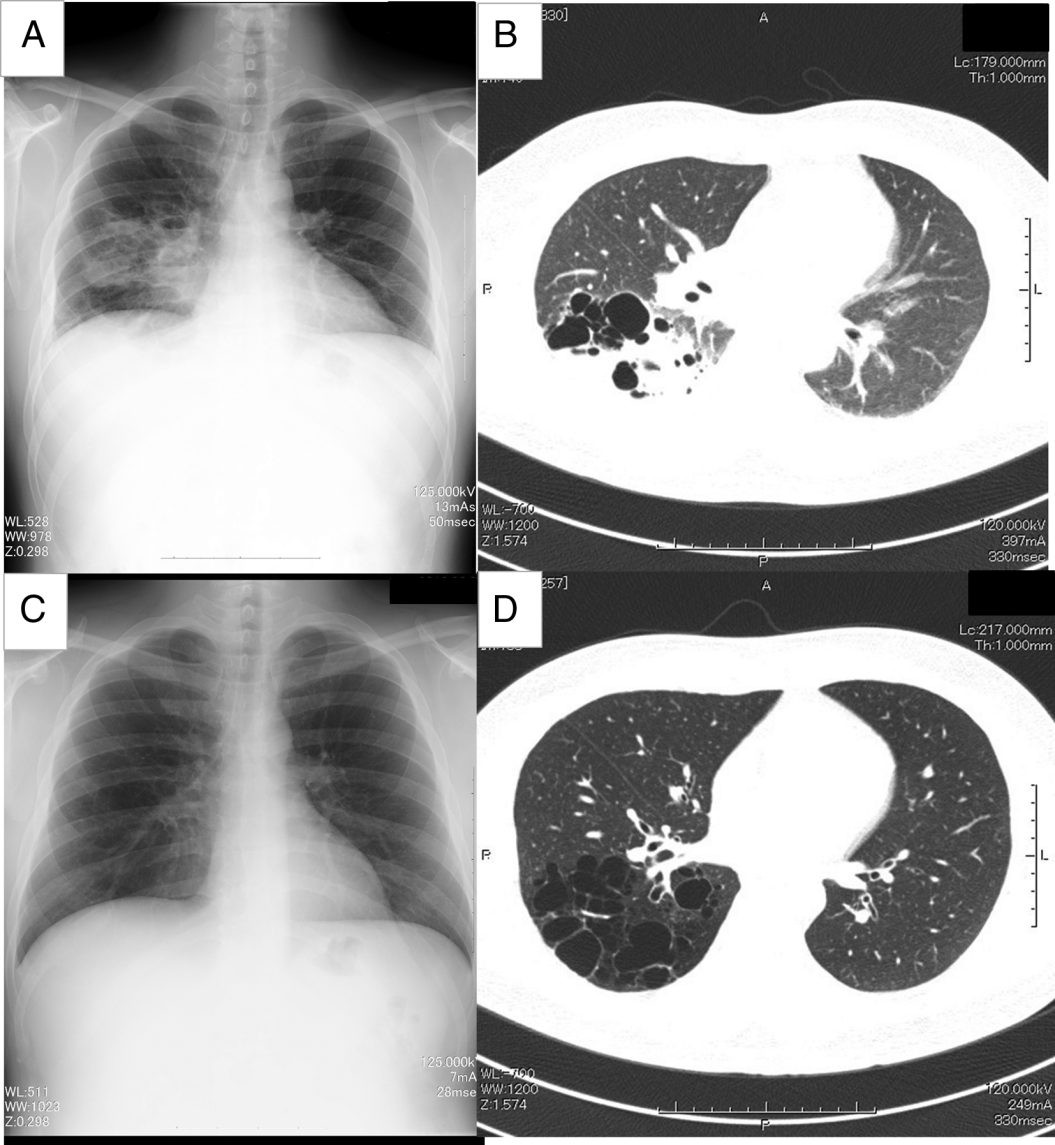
Case 2: (A) chest X‐ray at admission showed infiltrative shadows in the right lower lung field. (B) Chest CT scan at admission showed numerous cystic lesions and infiltrative shadows in the right lower lobe. After treatment for pneumonia, there was improvement in the above shadows on both (C) chest X‐ray and (D) chest CT scan.
